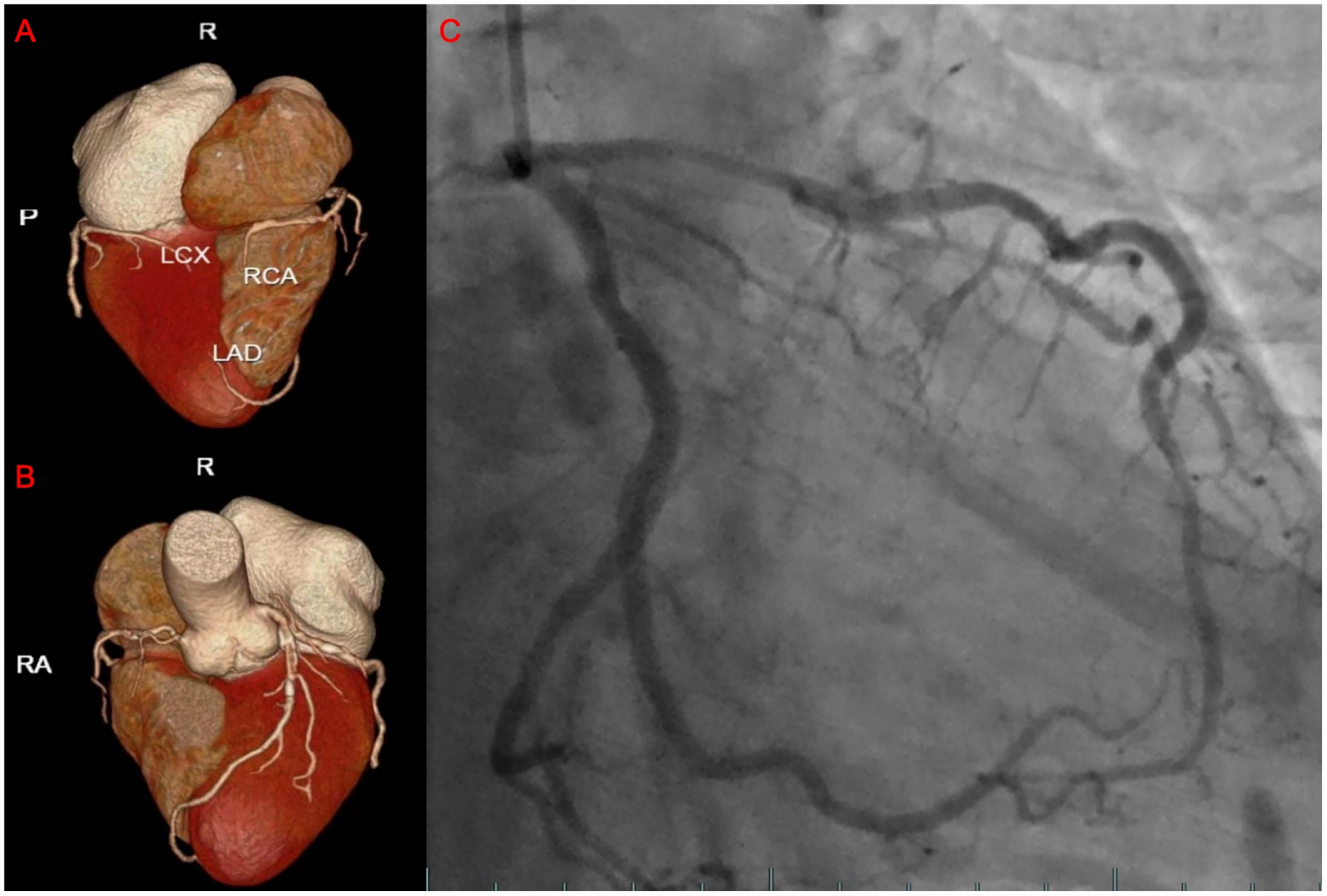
The results of the patient's coronary CTA and coronary angiography. (A,B) The three-dimensional reconstructed images of the patient's coronary arteries. (C) The images showing the passage of contrast agent through the patient's coronary arteries during angiography.
